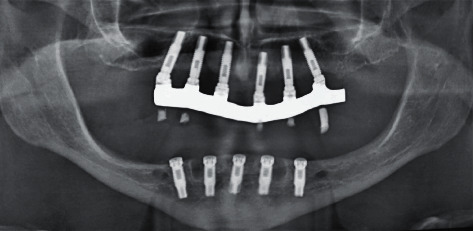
Control radiograph at 30 days.
